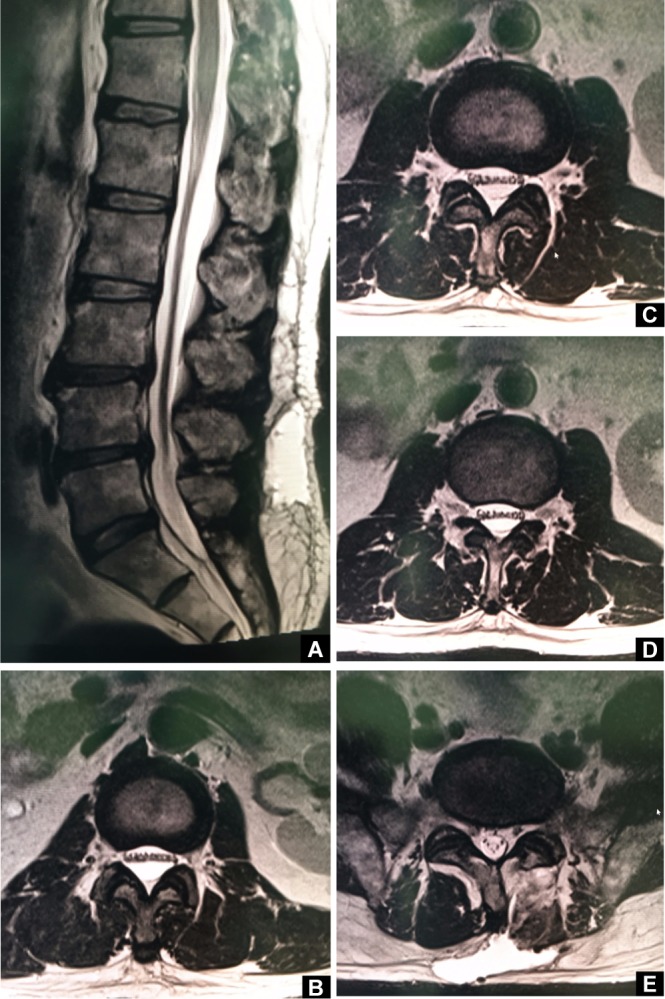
MRI images. (A) MRI sagittal cut depicting anterior translation of cauda equina from L1-L5 levels. (B) MRI axial cut at L1-L2 disc level showing unusual anterior linear arrangement of rootlets within a normally placed dura. (C) MRI axial cut at L2-L3 disc level. (D) MRI axial cut at L3- L4 disc level. (E) MRI axial cut at L4-L5 disc level showing pseudomeningocele with a fistulous tract.
